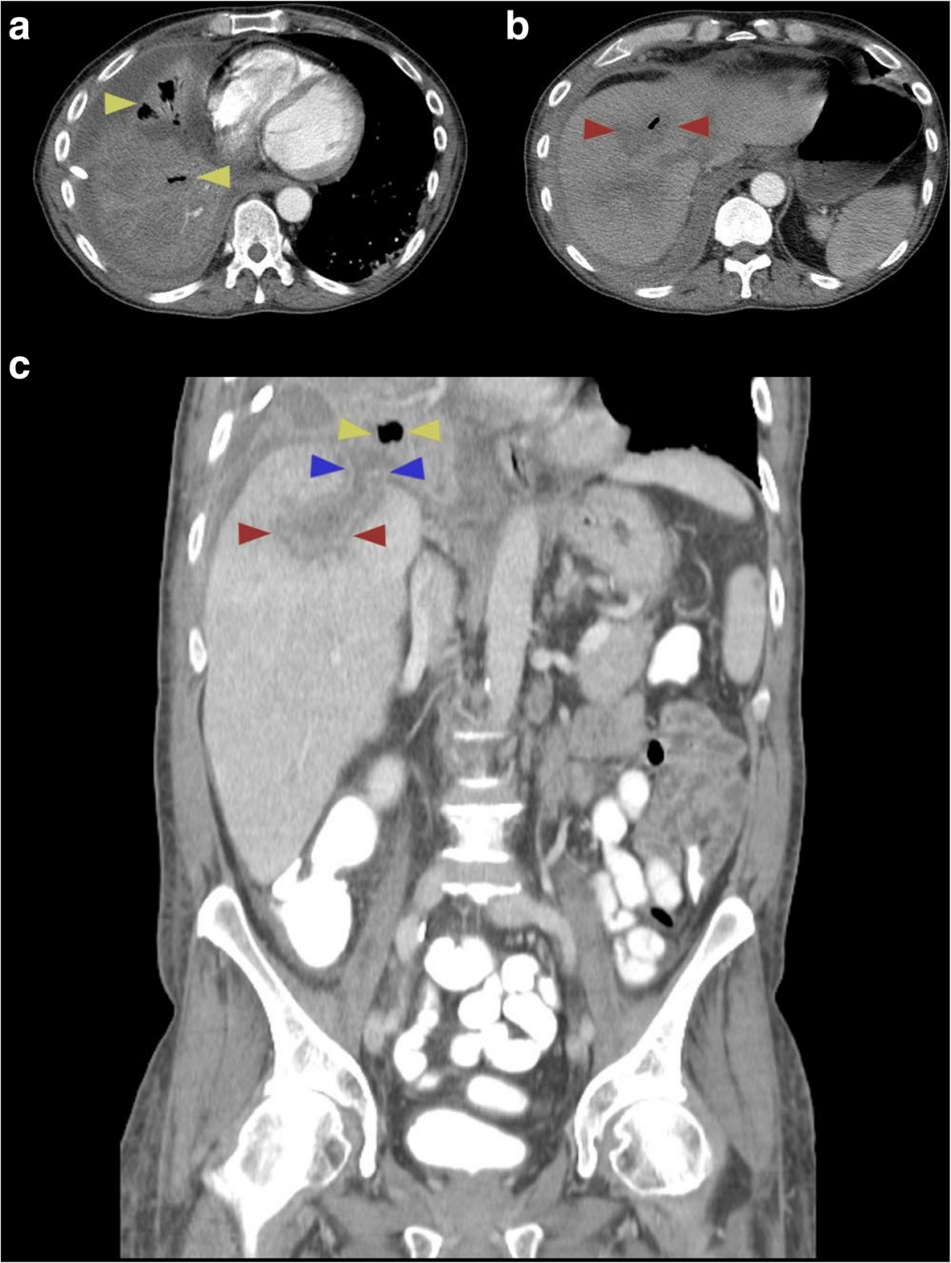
a Computed tomography thorax scan with contrast reveals multiple air-filled lung abscesses in the right lung lobe (*yellow arrows*). b The air-filled lung abscess is contiguous with a small, collapsed area of hypoattenuation within liver parenchyma with central air pocket in segment 8 of the liver (*red arrows*). c Computed tomography abdomen and pelvis scan with portal venous phase intravenous contrast clarified a continuous track (*blue arrows*) from a collapsed, peripherally enhancing liver abscess (*red arrows*) extending across the diaphragm and in continuity with right lower lobe lung abscesses (*yellow arrows*)
